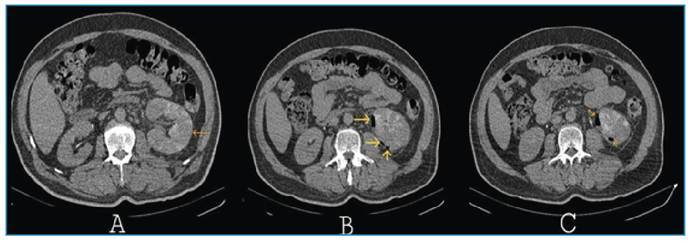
A: Computed tomography revealed a swollen area. B, C: Computed tomography revealed loculated gas collections in the lower left kidney.
